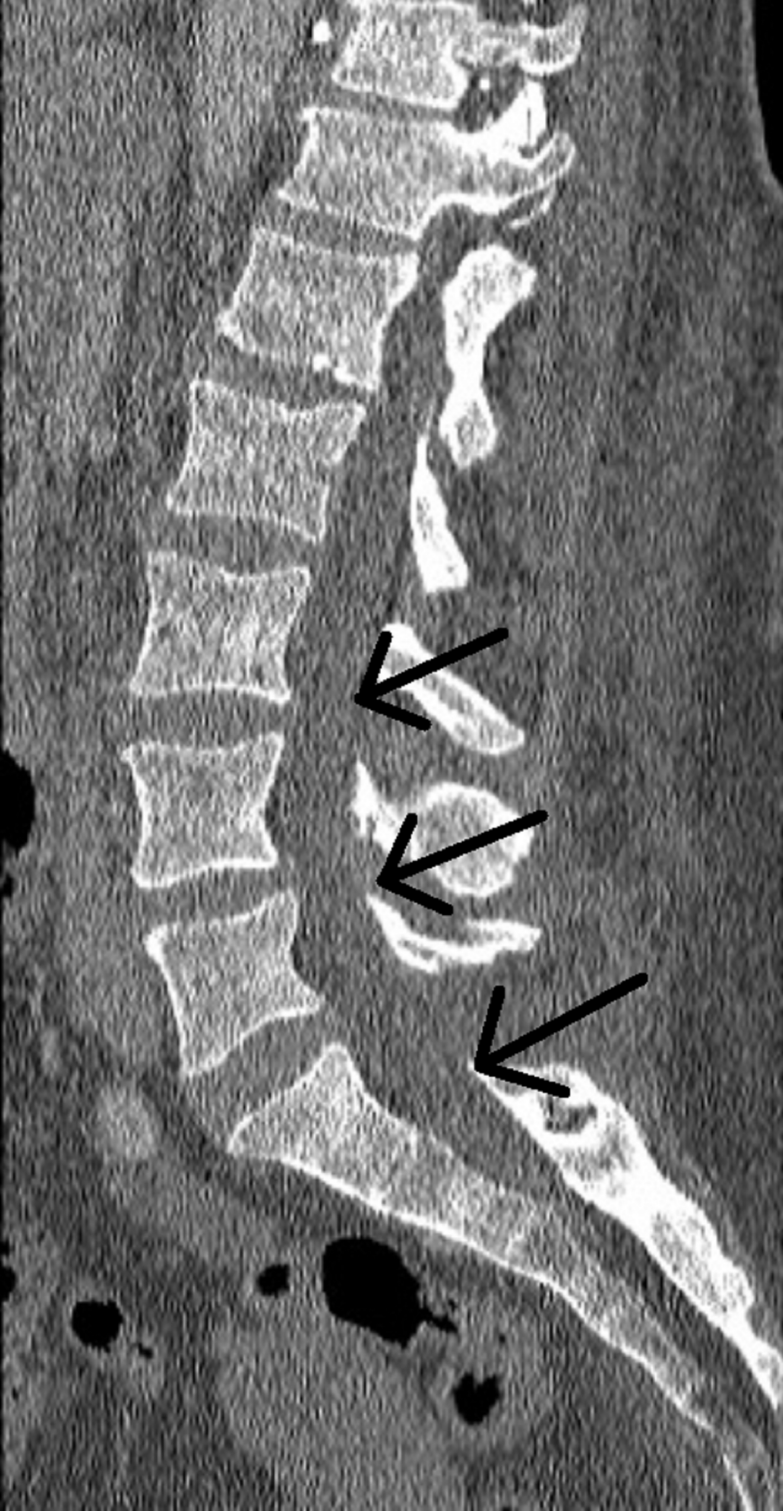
Lumbar CT with contrast at 16-day follow-up The image shows complete resolution of the epidural abscess and operative changes consistent with an L5/S1 laminectomy Black arrows indicate the former margins of the epidural abscess, now resolved CT: computed tomography
